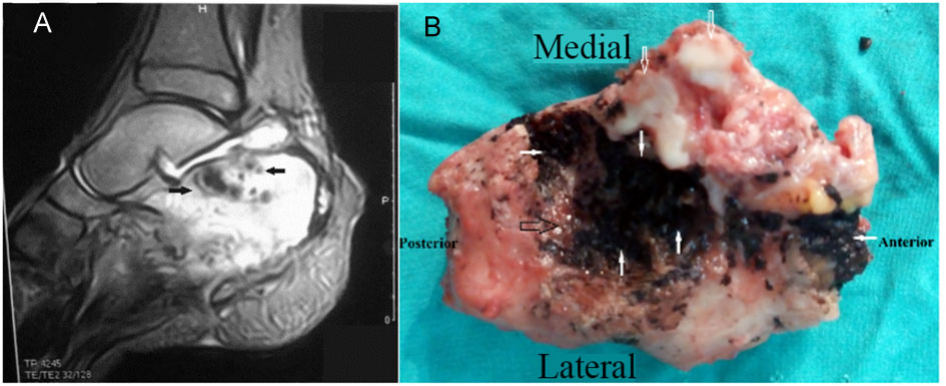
An adolescent boy from rural Egypt with eumycetoma osteomyelitis of calcaneus. (A) T2-weighted MRI shows dot-in-circle sign. (B) Operative image of superior calcaneus following calcanectomy shows extensive black grains and articular cartilage erosions (hollow white/black arrows). Adopted from El-Sobky et al.^9^
